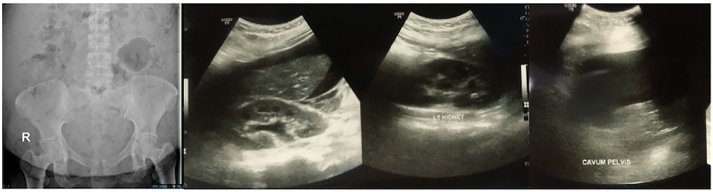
Radiology Examination, KUB X-ray, and FAST Results.
